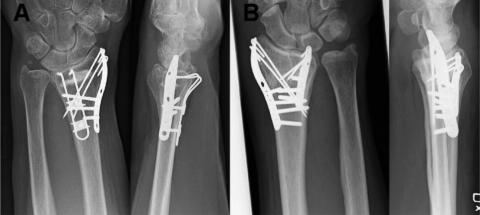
AP and lateral radiographs in two cases of distal radial fracture operated with the TriMed system. A. This patient was operated using a radial pin-plate and a volar buttress pin. Additional stability was achieved using Norian SRS bone substitute. B. In intraarticular fractures with an ulnar fragment, an ulnar pin-plate could be combined with the radial pin-plate.
